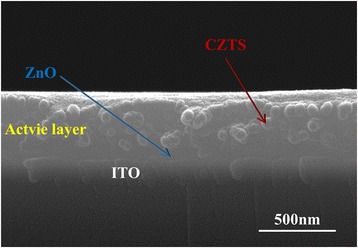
Cross-sectional SEM image of P3HT:PCBM:CZTS hybrid blend film deposited by ultrasonic spray
